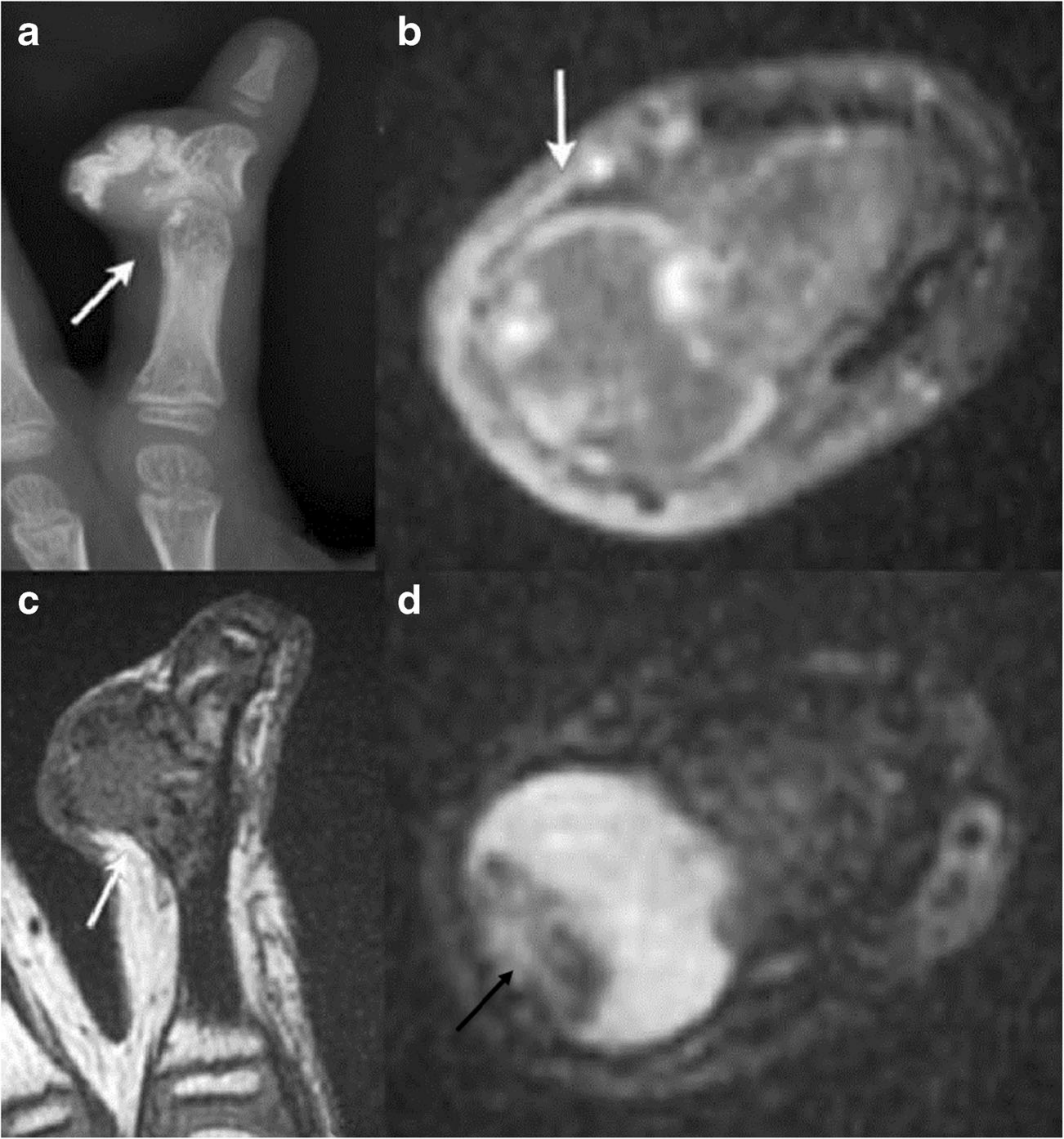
A 5-year-old boy with bizarre parosteal osteochondromatous proliferation at the middle phalanx of the right index finger. Antero-posterior radiograph of the right index finger a demonstrates a well-defined mass with amorphous calcifications (*arrow*) adjacent to the otherwise intact cortical surface of the middle phalanx. No medullary continuity is demonstrated between the lesion and underlying bone. Axial T1 post-contrast image b shows irregular peripheral contrast enhancement of the lesion without surrounding soft-tissue invasion (*arrow*). The lesion is hypointense on coronal T1 c and mostly hyperintense with few hypointense foci on axial T2 d (*black arrow*)Dysplasia epiphysealis hemimelica (DEH):This is also known as Trevor’s or Trevor–Fairbank disease and is a rare developmental disorder of the skeleton characterized by asymmetric osteochondral overgrowth of a medial or lateral epiphysis or epiphyseal equivalent [15]. This most frequently affects the knee and ankle, presents as a hard, painless mass adjacent to the affected joint with pain/discomfort developing at a later stage. The medial aspect of the epiphysis is more commonly affected than the lateral aspect.On radiographs, asymmetric cartilaginous overgrowth may be seen with multiple ossific centres and a stippled, irregular or dense pattern of epiphyseal chondral calcification. Affected epiphysis is usually larger than that of the contralateral normal limb. Associated metaphyseal widening and remodelling may occur [15].MR is the modality of choice for identifying dimensions of the cartilage mass, neurovascular or ligamentous involvement, associated complications such as bursitis and arthritis and distinction from other pathology. The typical imaging characteristics of cartilage are seen on both noncontrast and post-contrast MR. With increasing lesion maturity, MRI demonstrates cortical and medullary coalescence between the ossification centre of the lesion and the adjacent epiphysis — the marrow signal intensity parallels that of the normal bone (Fig. 8). Signal voids on T1/T2 represent foci of dense calcification within the marrow [15].
